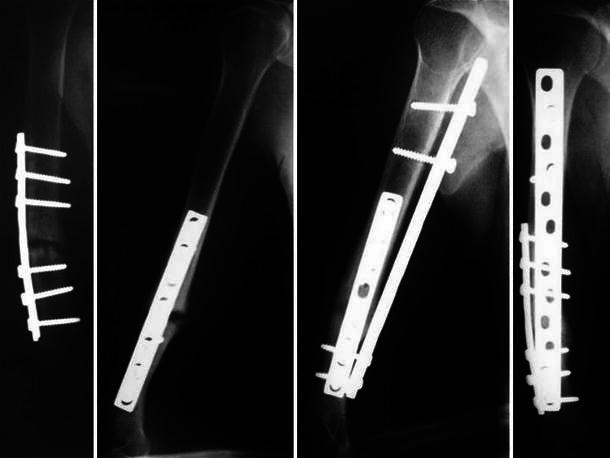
X-ray images (frontal and lateral views) demonstrate atrophic nonunion instability signs—varus alignment and initial plate bending (*left*). Six weeks postoperatively with bone formation in the nonunion site (*right*)
